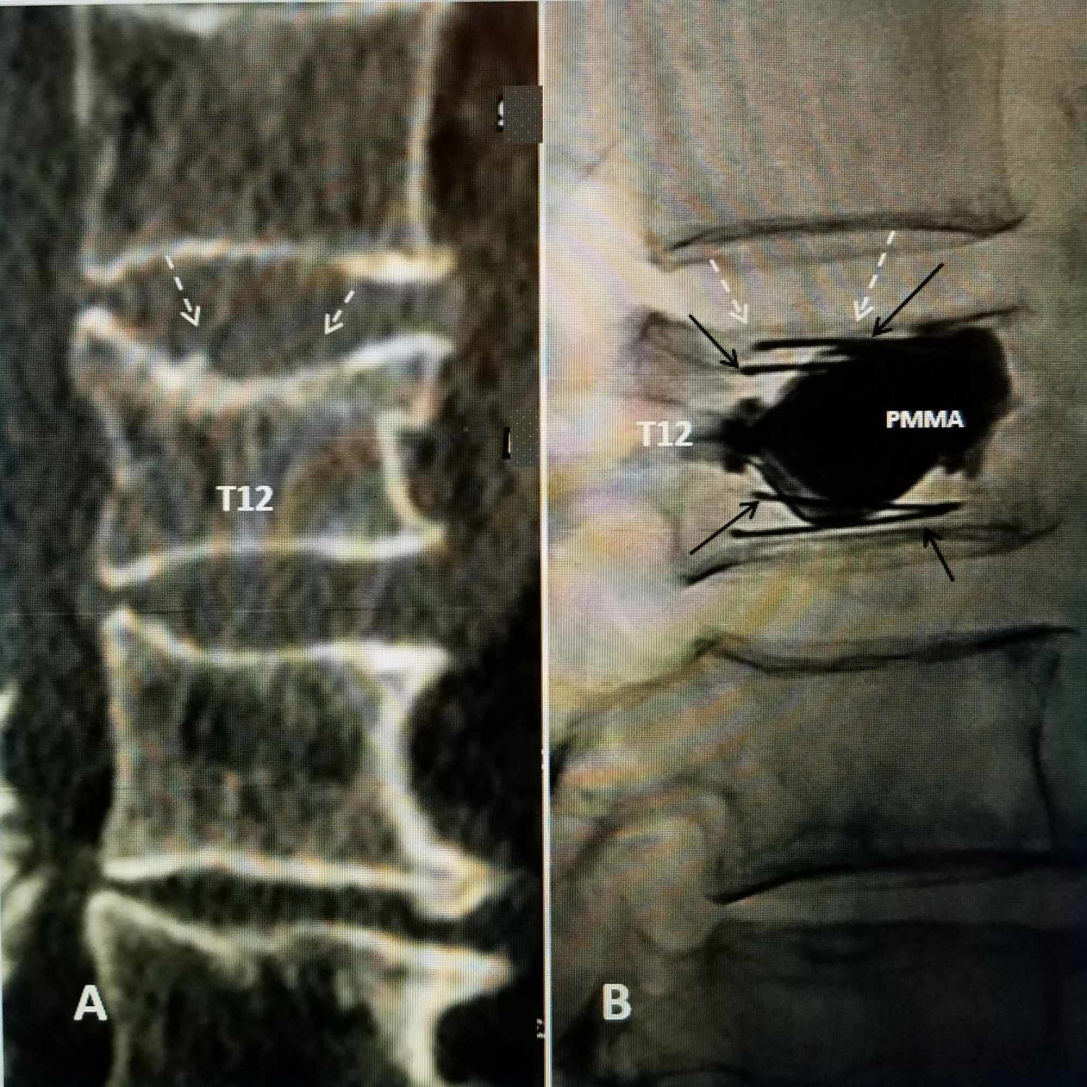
T12 fracture with two SpineJack implants placed in vertebral body with re-expansion of the superior endplate A: Pre-operative sagittal CT scan showing compression fracture of the superior endplate of T12 (dashed white arrows). B: Post-procedure digital fluoroscopic lateral image showing almost complete re-expansion of the superior endplate (dashed white arrows). The struts of the two parallel SpineJack 5.0-mm implants are clearly seen extending under the length of both superior and inferior endplates (solid black arrows). The cement is confined between the struts and the two implants (PMMA). PMMA, polymethylmethacrylate
